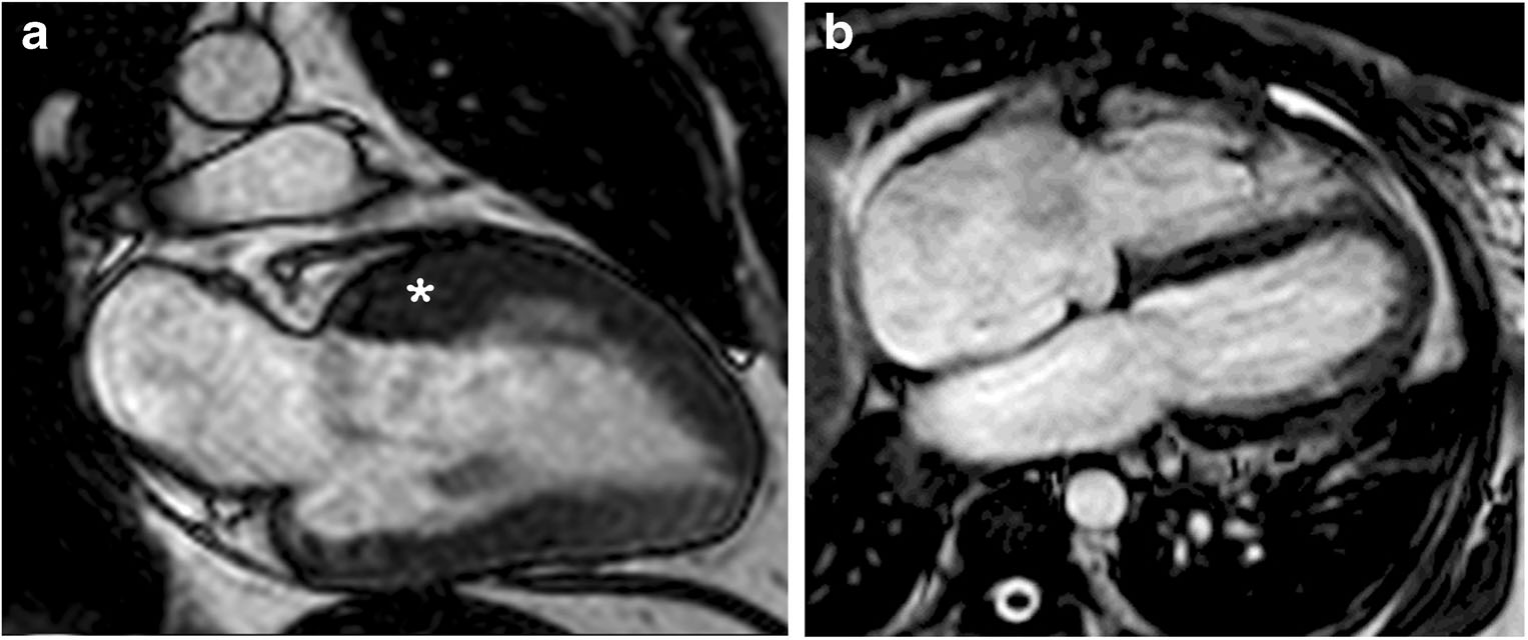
End stage HCM with restrictive pattern. 77-year-old man who presented with several months of intermittent exertional dyspnea and asymmetric HCM. He presented to the emergency room 7 years later with markedly worsening dyspnea and decompensated heart failure. a Two-chamber SSFP cine MR image performed at initial diagnosis shows asymmetric anterior hypertrophy (asterisk) with an ejection-fraction of 60%. b, Four-chamber SSFP cine MR image performed 7 years later demonstrates a small LV cavity, atrial cavities enlargement, regression of wall thickness and a reduced ejection-fraction of 37%
